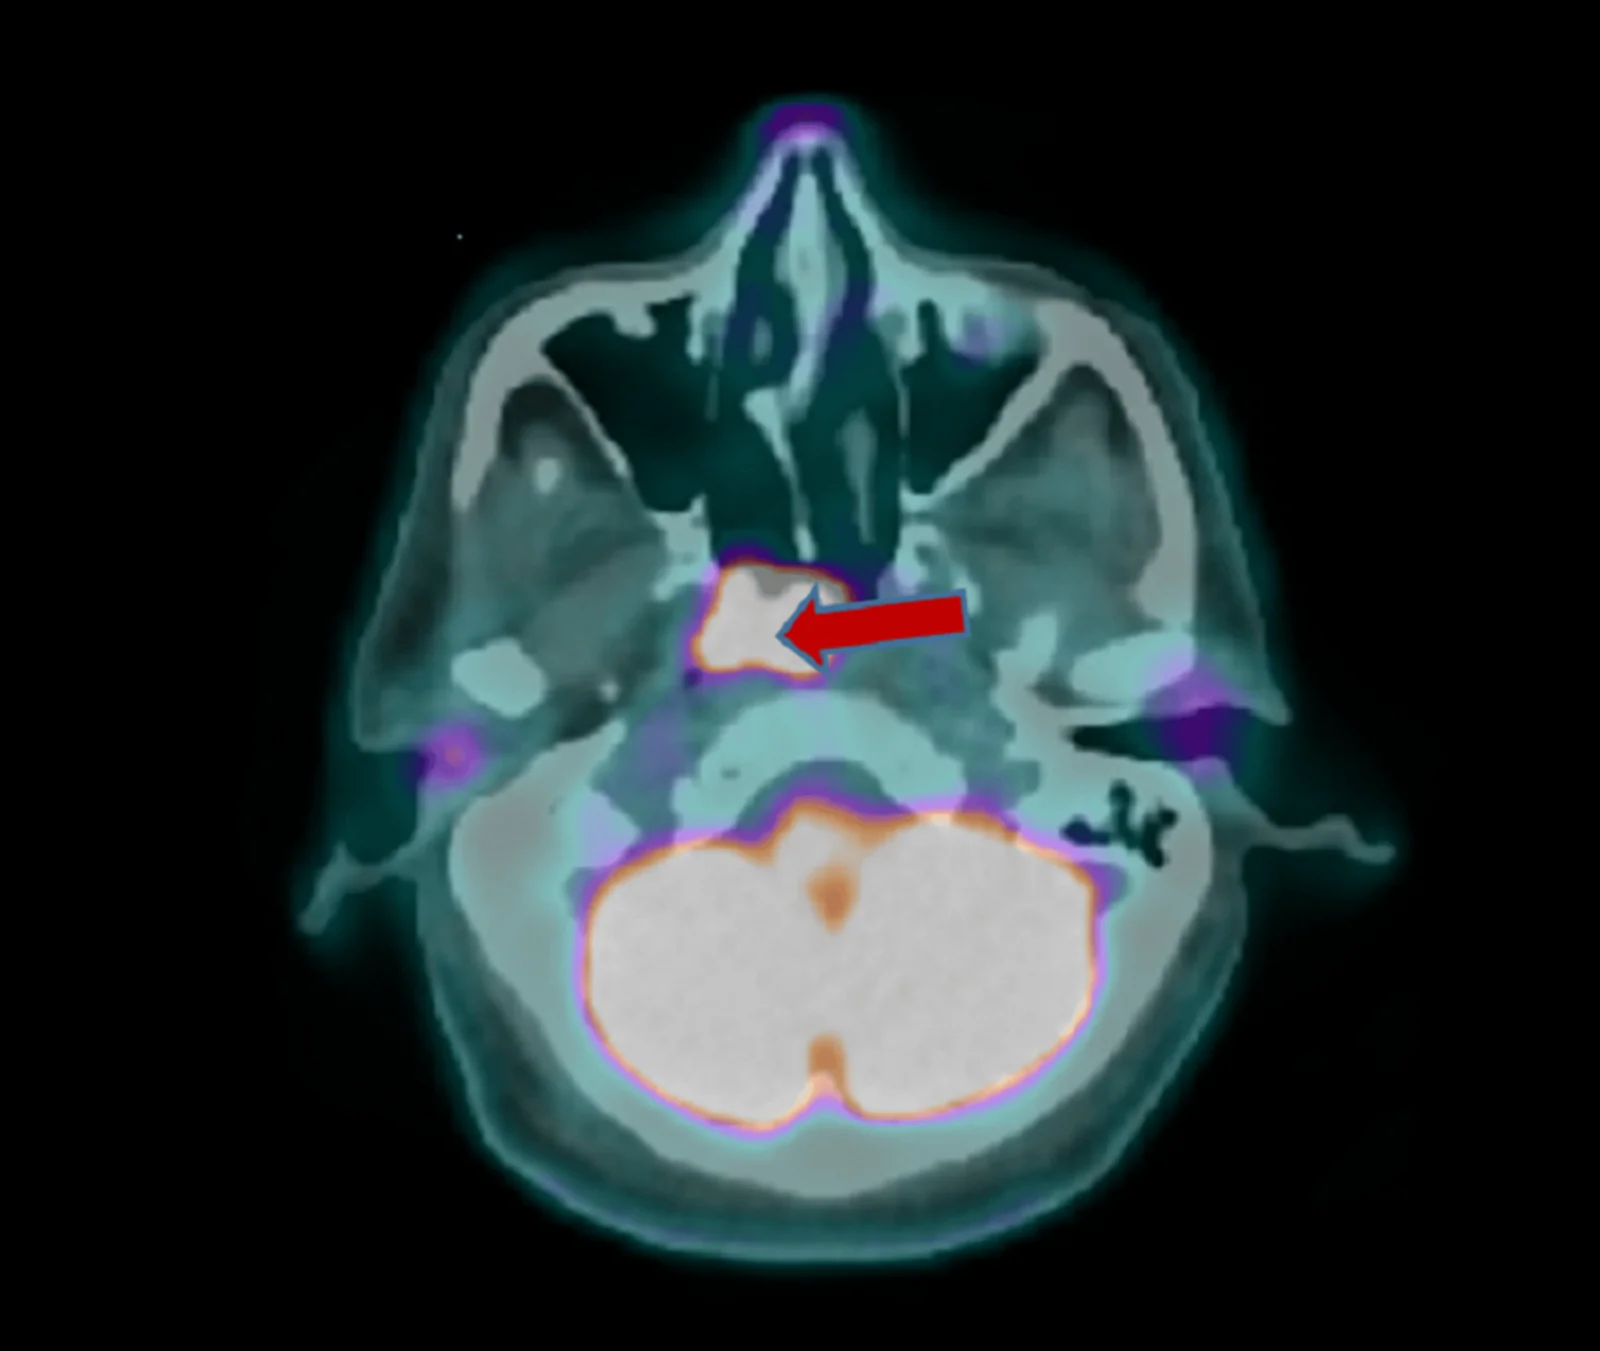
Positron emission tomography computed tomography showing the nasopharyngeal tumor before treatment (arrow)
